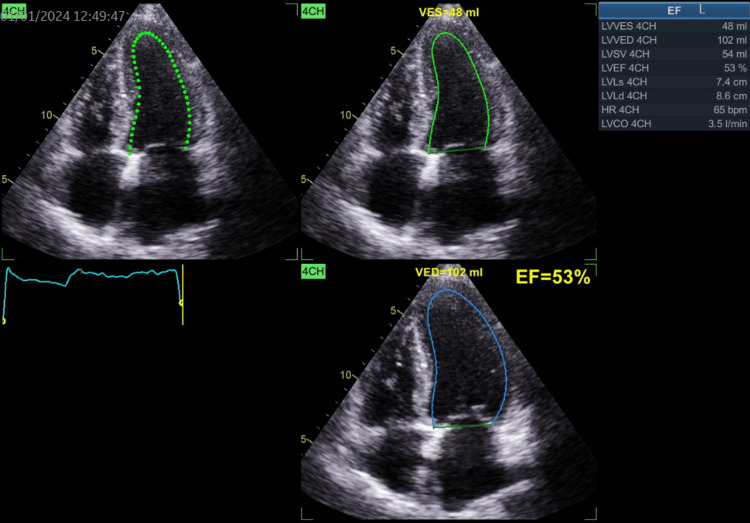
Left ventricular ejection fraction recorded at 53% from the apical four chambers view. EF: Ejection fraction; VED: Volume at end diastole; VES: Volume at end systole.
